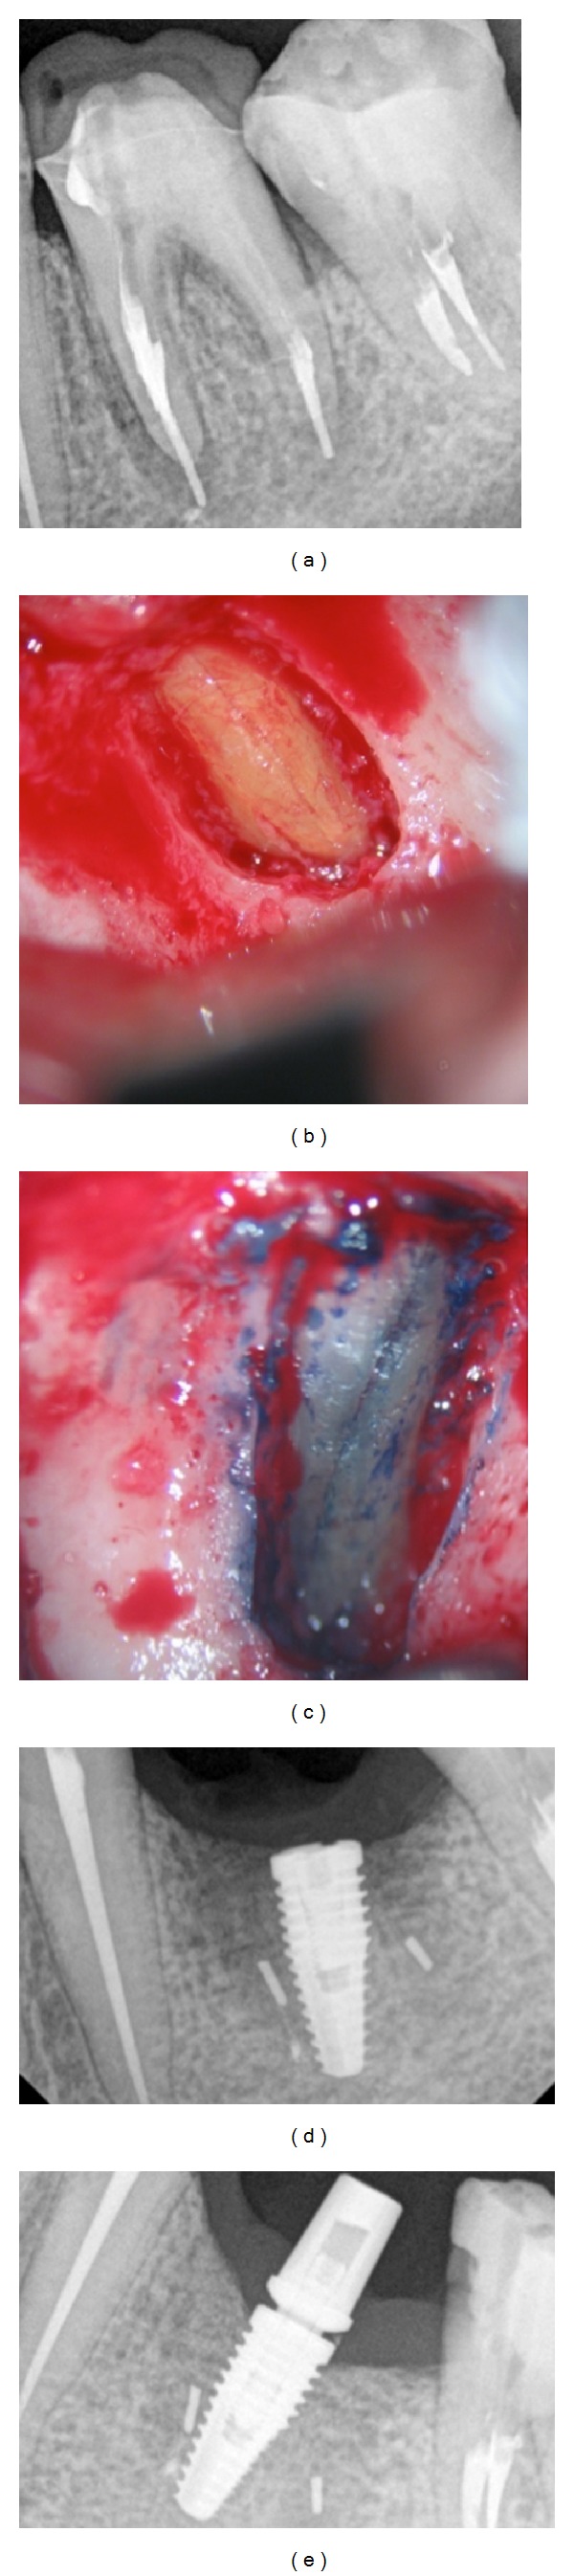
Initial radiograph of the first molar. Observe the extravasation of the filling material in the mesial root (a). Clinical aspect of the mesial root showing the vertical root fracture and bone fenestration (b). Application of dye (SableSeek, Ultradent) confirming the presence of RVF (c). Radiograph aspect after immediate implant placement (Neodent, 10.0 × 4.3) (d). Radiograph aspect 4 months after the immediate implant installation with the placement of temporary crown (e).
